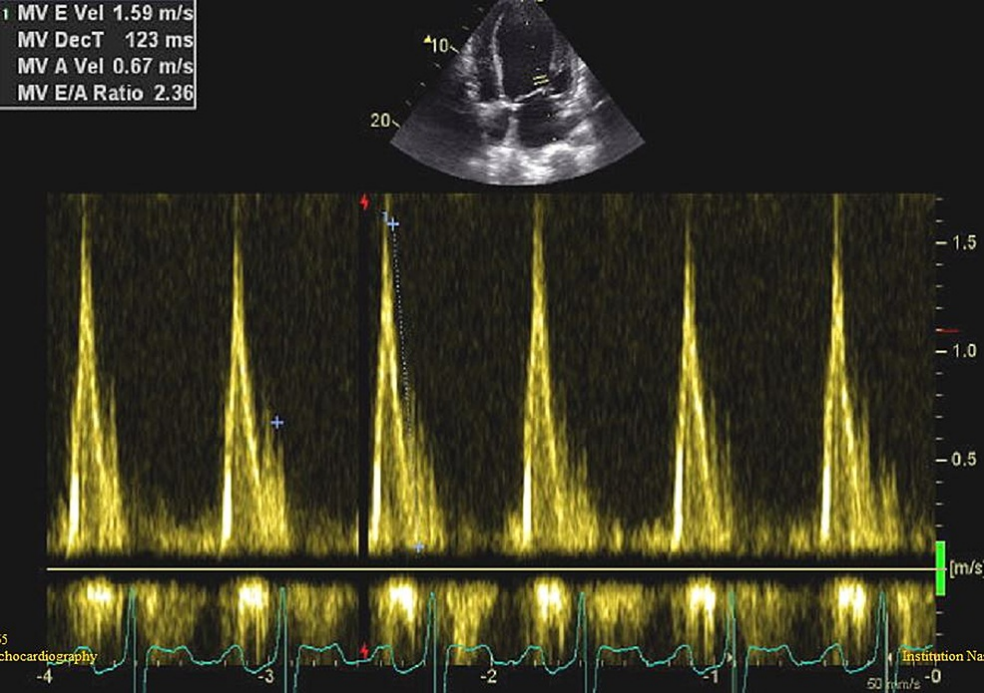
Pulse wave Doppler image showing signs of restrictive pattern with high E waves velocities.
